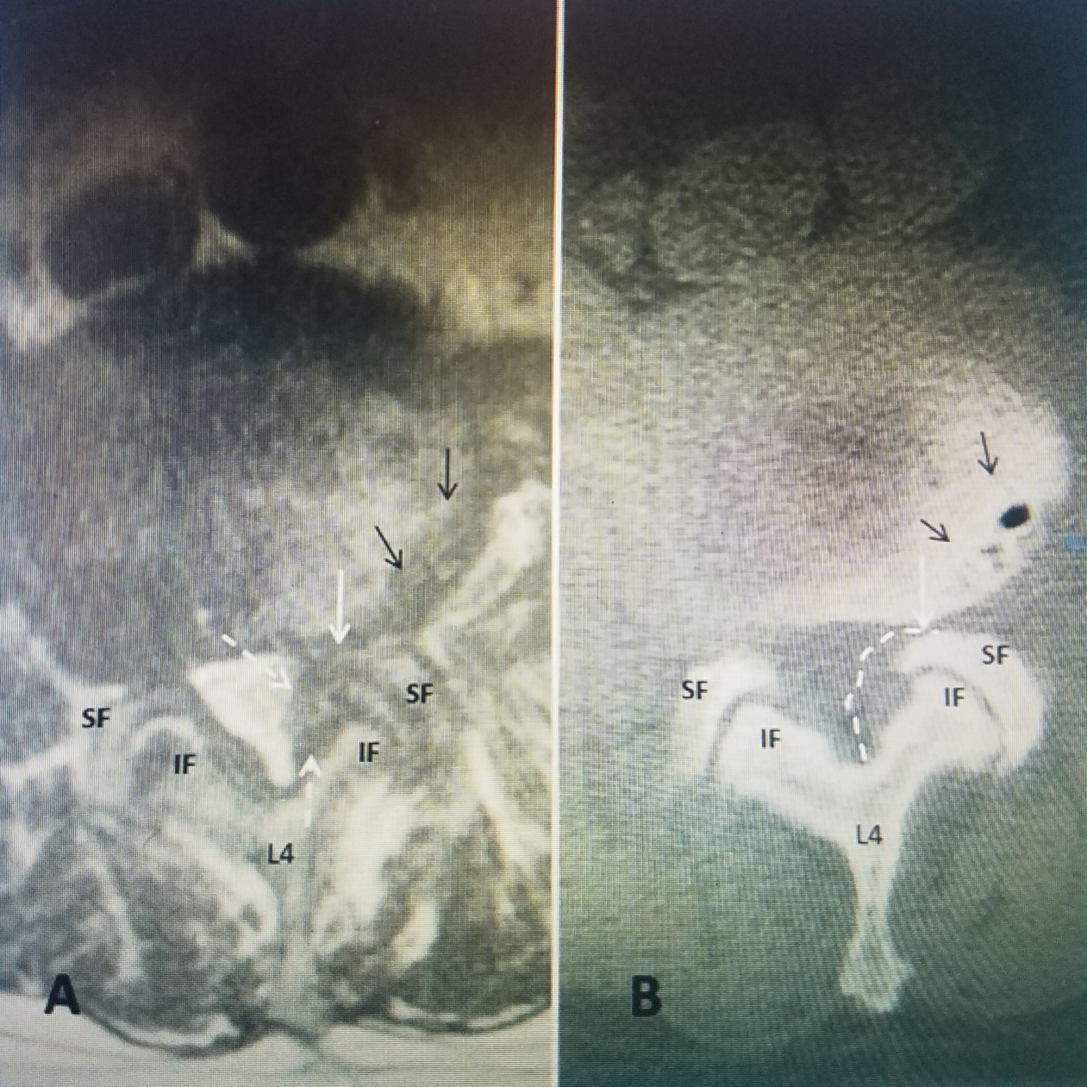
Matched axial cuts at L4-5 in the same patient comparing MRI to CT scan. A: Axial T2 MRI at L4-5 demonstrates the lateral recess stenosis on one side (dashed white arrows) compressing approximately 50% of the lateral spinal canal almost to the midline. The inferior facet (IF) of L4 and superior facet (SF) of L5 are identified. The neural foramina is compressed (solid white arrow) and there are also irregular subchondral endplate cysts in the vertebral body on the same side (solid black arrows). B: Axial CT scan in the same patient made at the same level. Compared to the MRI, the CT scan demonstrates the relationship of the superior facet (SF) to the stenotic foramina (solid white arrow). The soft tissue stenosis seen on MRI in 'A' but not on CT scan is marked(dashed white arrow). The inferior facet (IF) of L4 and the superior facet of L5 can be seen compressing the neural foramina (solid white arrow). Comparing the MRI to the CT demonstrates that the compression seen on the MRI is secondary to soft tissue hypertrophy rather than bony facet overgrowth.
